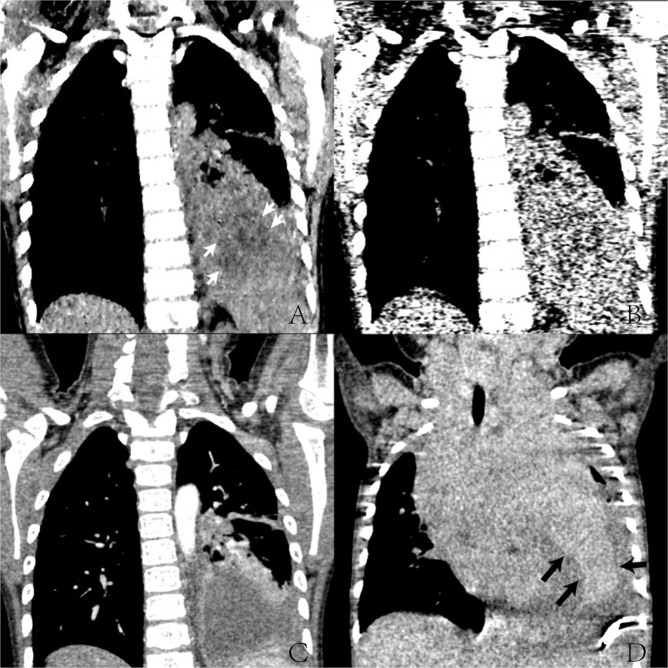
The reconstructed image of coronal section images. (A–C) 3 years old boy with pneumonia. (A) Low dose (0.09 mSv) MBIR image (LD-MBIR), (B) Low dose ASIR50% images (LD-ASIR) and (C) routine dose CECT ASIR50% image, radiation dose was 1.54 mSv. LD-MBIR images showed a patch of low density in the center of consolidation (white arrow head), but the boundary was blur, which was confirmed by the routine dose CECT image as a necrotic lesion, that LD-ASIR was not able to display it. (D) Another 2 years old boy with Lymphoma. Routine dose non enhanced CT images (RD-ASIR), radiation dose was 1.38 mSv, which can display different density well, the boundary was clear (black arrow).
